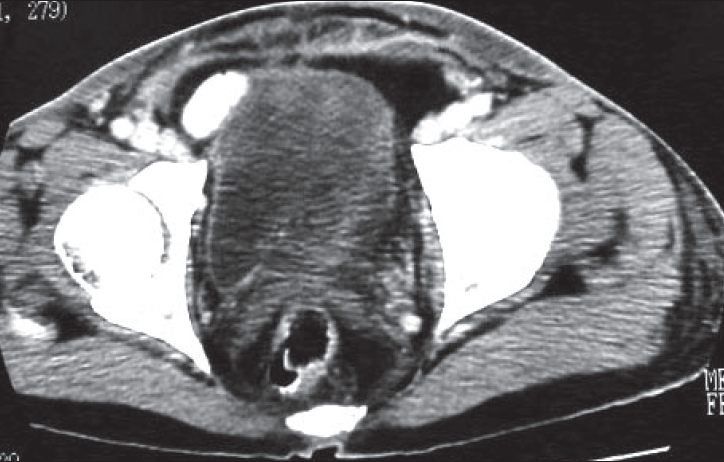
CT scan of the lower abdomen
